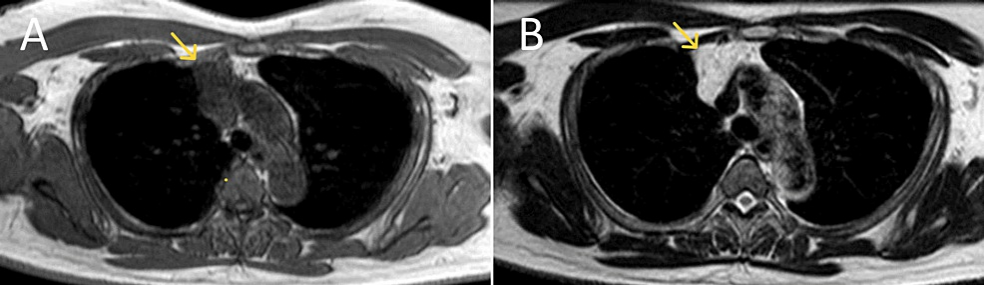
T1-weighted imaging (T1WI) and T2-weighted imaging (T2WI) MRI The mass appeared isointense on T1WI (A) and hyperintense on T2WI (B), with no observed flow voids.
